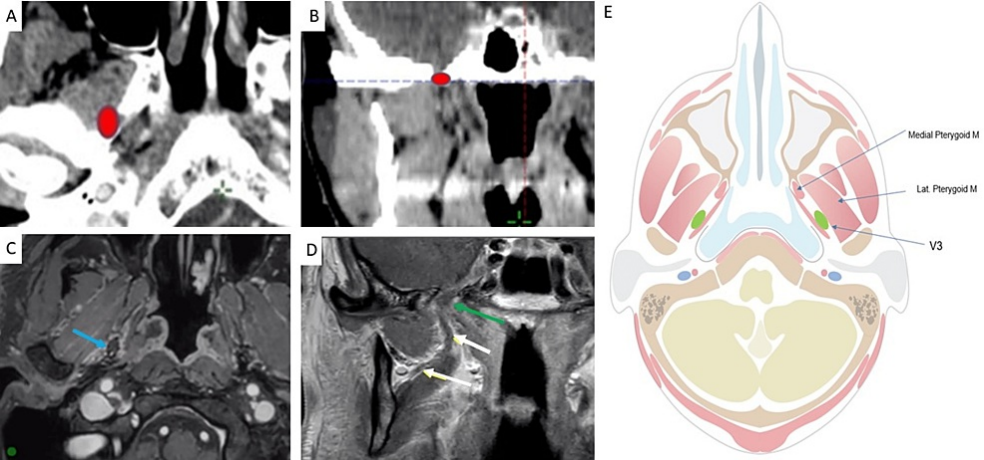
Anchor point at the level of the foramen ovale A: Location of V3 (red) on axial CT B: Location of V3 (red) on corresponding coronal CT slice C: Location of V3 (blue arrow) on axial fat-suppressed contrast-enhanced T1-weighted (T1W) MRI D: Location of foramen ovale (green arrow) and V3 medial to lateral pterygoid muscle (white arrows) on axial fat-suppressed contrast-enhanced T1 MRI E: Anatomic design, created by author Anastasia Tjan
